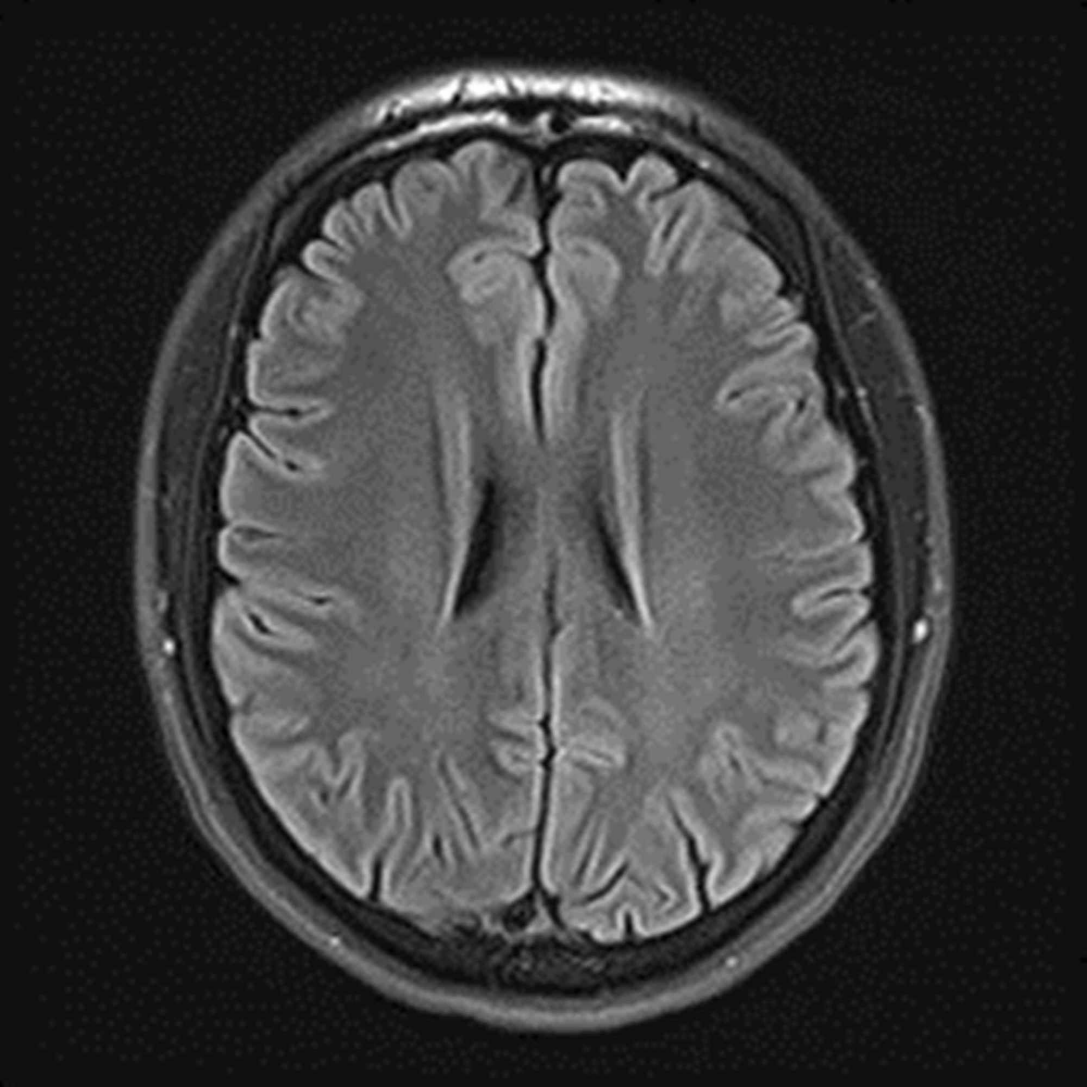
MRI-3T brain non-contrast showing normal brain with no evidence of demyelination.
